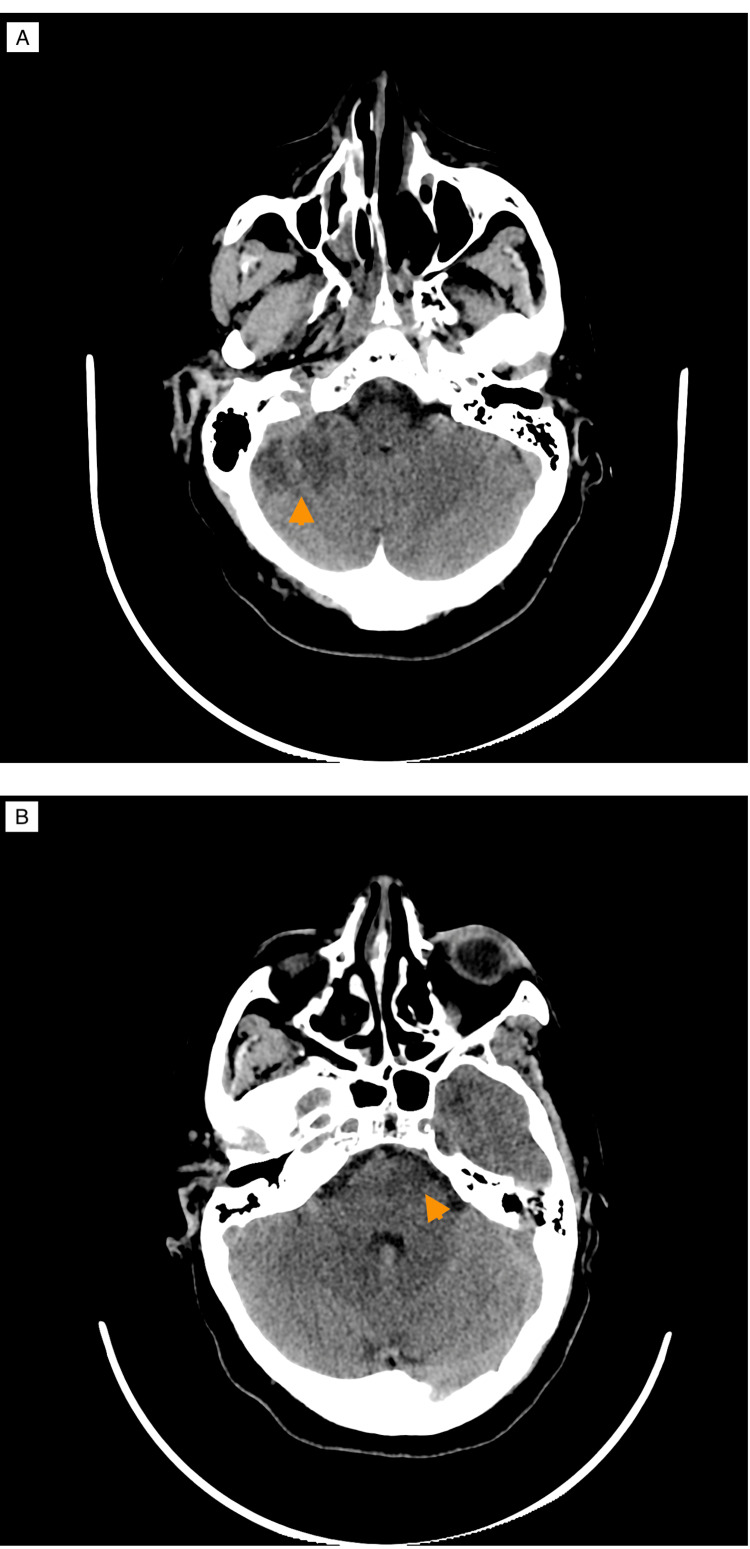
Head computed tomography scan depicting resultant ischemic lesions from basilar artery occlusion. (A) shows a hypodense right cerebellar lesion corresponding to the territory supplied by the right anterior inferior cerebellar artery (arrowhead). (B) shows a hypodense left pontine lesion corresponding to the territory supplied by the basilar artery.
